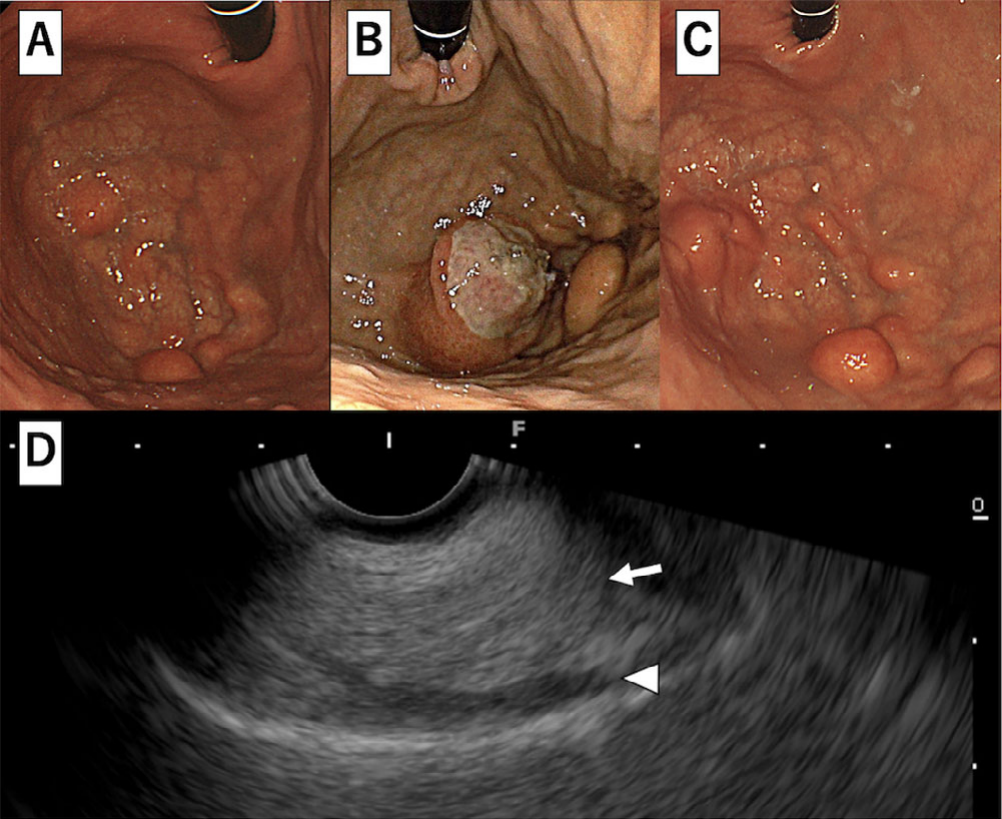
Esophagogastroduodenoscopy in X‐3 reveals multiple submucosal tumor‐like elevations (8–15 mm) in the greater curvature of the stomach (A). The submucosal tumor is significantly enlarged, with the largest reaching 3 cm in diameter and showing self‐destruction at the top (B). In year X + 2 months, the ulcer at the top disappeared (C). Endoscopic ultrasonography with a 7.5 MHz convex probe reveals a hyperechoic mass in the third layer of the stomach wall (D). The tumor is indicated by an arrow, and the muscularis propria layer is indicated by an arrowhead.
